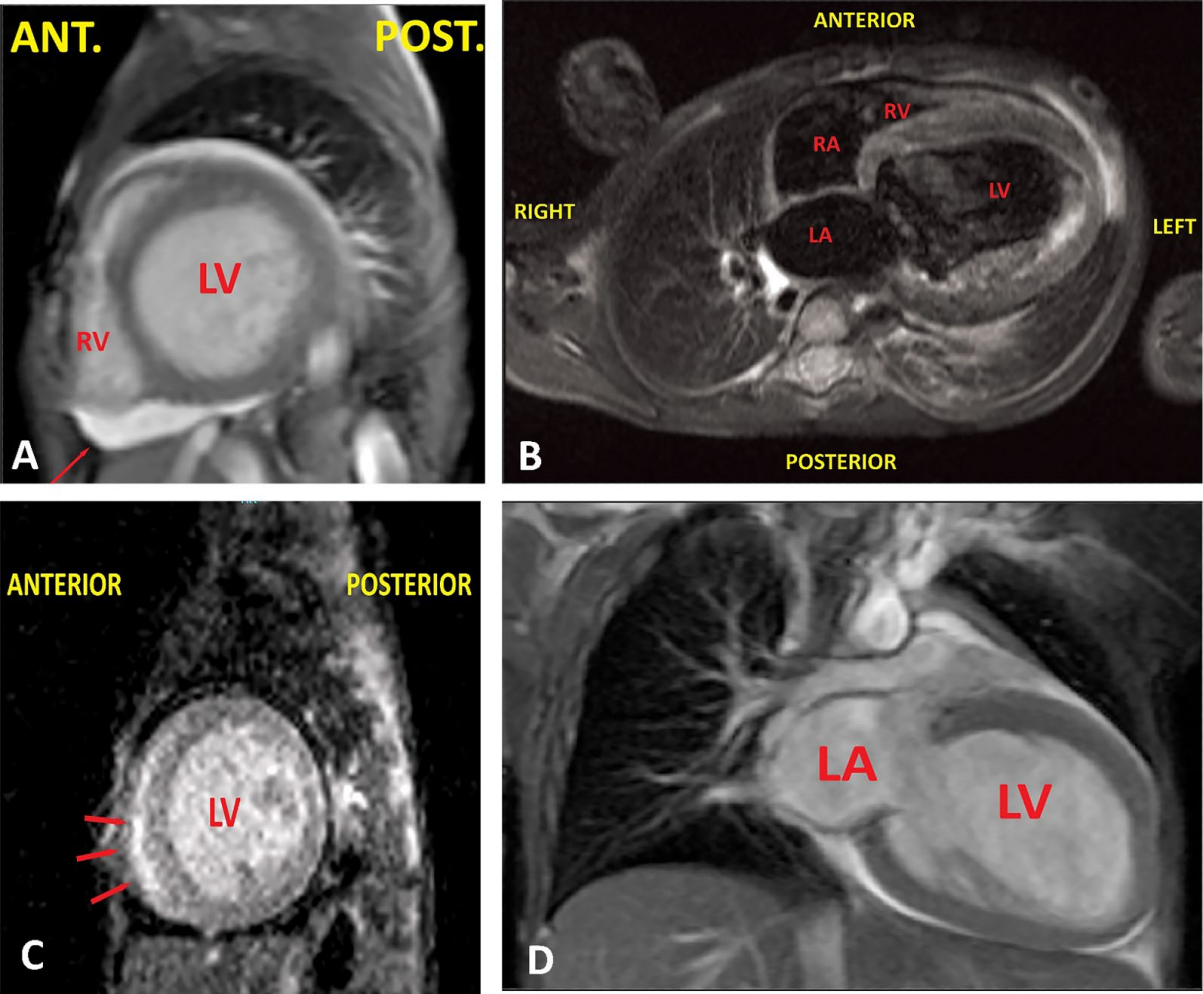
Cardiac magnetic resonance imaging of the patient. A Pericardial effusion, B indicates diffuse myocardial edema, C depicts late gadolinium enhancement, and D shows a severe enlargement of the left ventricle
